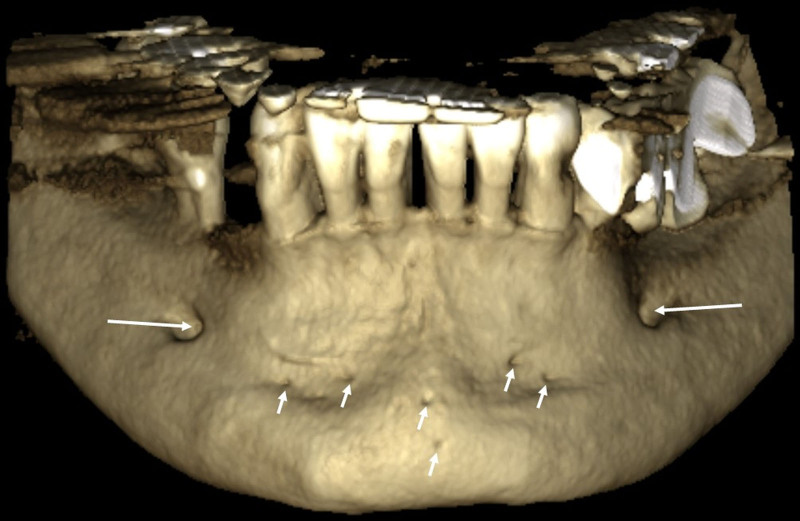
3D reconstructed CBCT image. Labial foramina (short arrows), bilateral mental foramen (long arrows). 3D = three-dimensional, CBCT = cone beam computed tomography.
